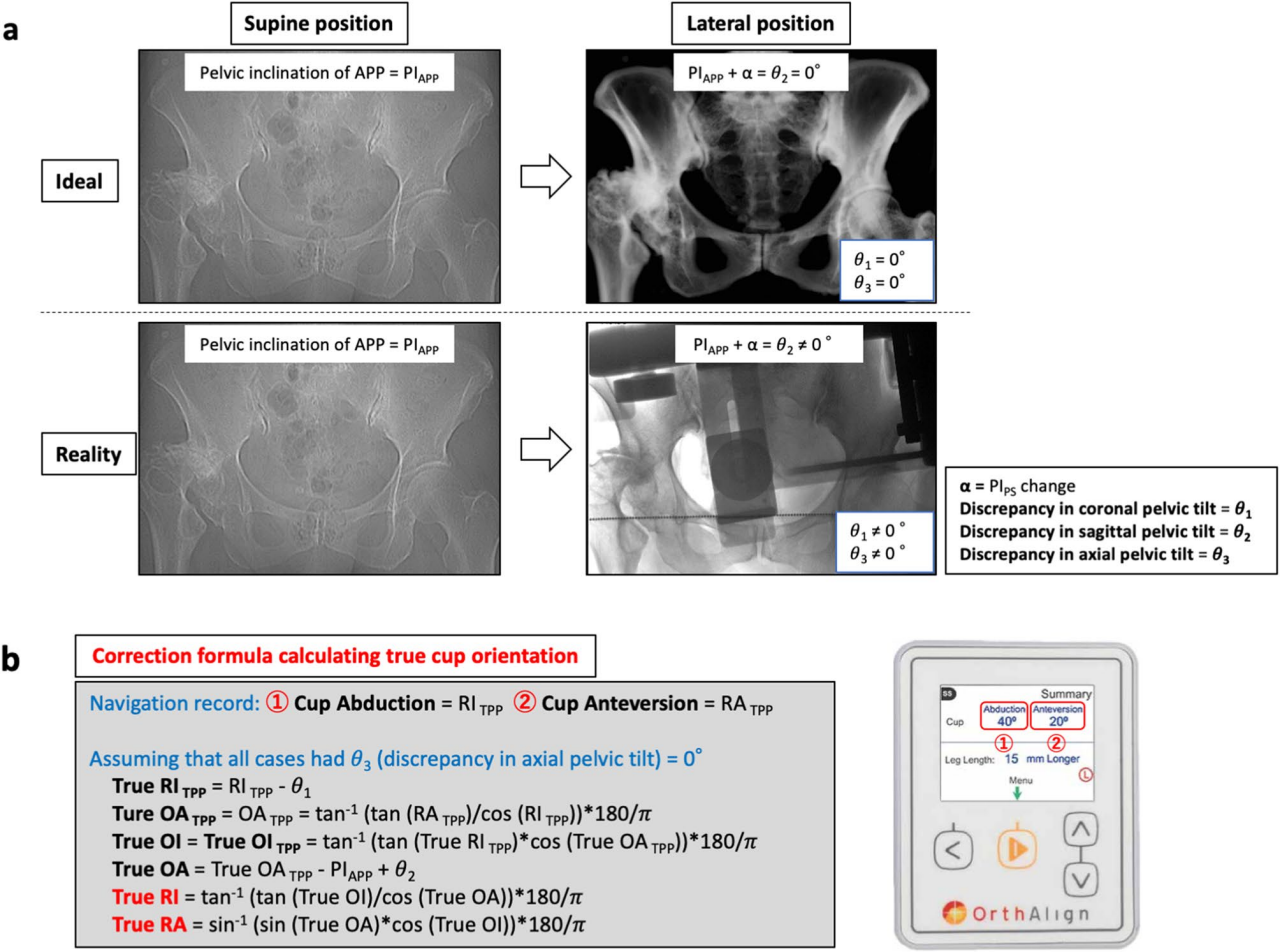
Correction formula calculating true cup orientation based on the discrepancy in pelvic tilt, as measured by TPP images. (a) Although the APP should ideally be parallel to the TPP, pelvic tilt discrepancies were observed in many cases in reality. (b) We created a correction formula to calculate the true cup orientation based on the pelvic tilt discrepancies (θ_1_ and θ_2_) measured by the TPP images. APP, anatomic pelvic plane; PI_PS_, pelvic inclination of plane formed by pubic symphysis and sacral promontory in supine or lateral position; RI, radiographic inclination; RA, radiographic anteversion; OI, operative inclination; OA, operative anteversion; TPP, table parallel plane; RI_TPP_, RI based on the TPP; RA_TPP_, RA based on the TPP; OI_TPP_, OI based on the TPP; OA_TPP_, OA based on the TPP.
